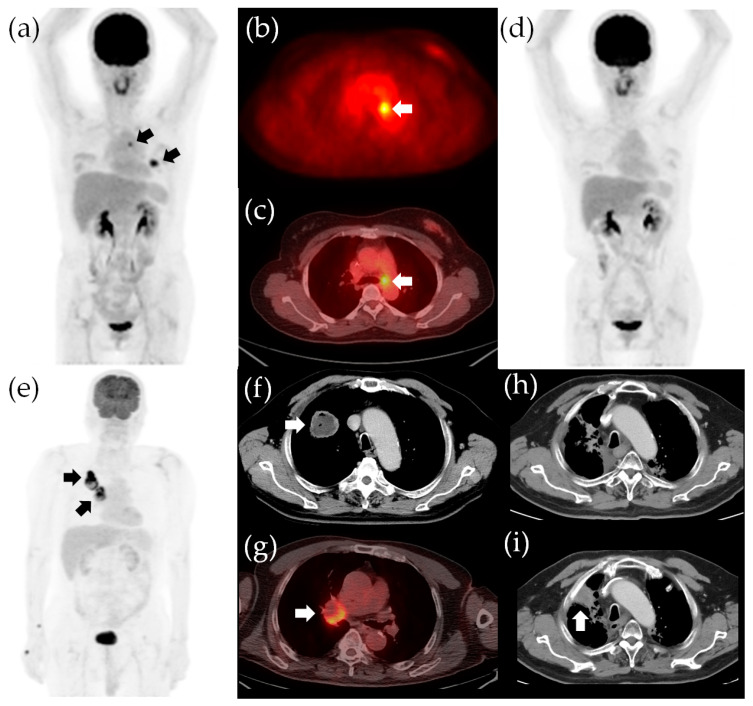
Survival stratification according to the independent risk factors in our study. The ^18^F-FDG PET/CT images for a 50-year-old woman with adenocarcinoma in the left upper lobe and subaortic nodal metastasis, indicated by arrows in the panels (a–c). The clinical staging was cT2aN2M0, stage IIIA. The total TLG was 14.9 and the ECOG status was 0. The patient had no poor survival risk factor (hazards were both 1 for an unfavorable OS and poor PFS) and she underwent lobectomy of the left upper lobe and mediastinal lymph node dissection. The pathological staging was pT2aN2M0, stage IIIA. She underwent adjuvant chemotherapy and is now alive without recurrence (d). The OS and PFS were 116 and 115 months, respectively. A 75-year-old man with adenocarcinoma in the right lower lobe and ipsilateral hilar nodal metastasis, indicated by arrows in the panels (e–g). The clinical staging was cT2bN1M0, stage IIB. The total TLG was 246.6 (>81) and the ECOG status was 1 (the hazards for unfavorable OS and poor PFS were 16.8 and 3.3, respectively). The patient received definitive CCRT (2 Gy/fraction daily to a targeted dose of 66 Gy) and marked tumor shrinkage was observed (h). However, the patient experienced progression of the primary tumor 15.9 months after definitive CCRT, indicated by the arrow in the panel (i). The patient eventually died of lung cancer progression, with an OS of 24.0 months and PFS of 15.9 months. TLG, total lesion glycolysis; OS, overall survival; PFS, progression-free survival; CCRT, concurrent chemoradiotherapy.
